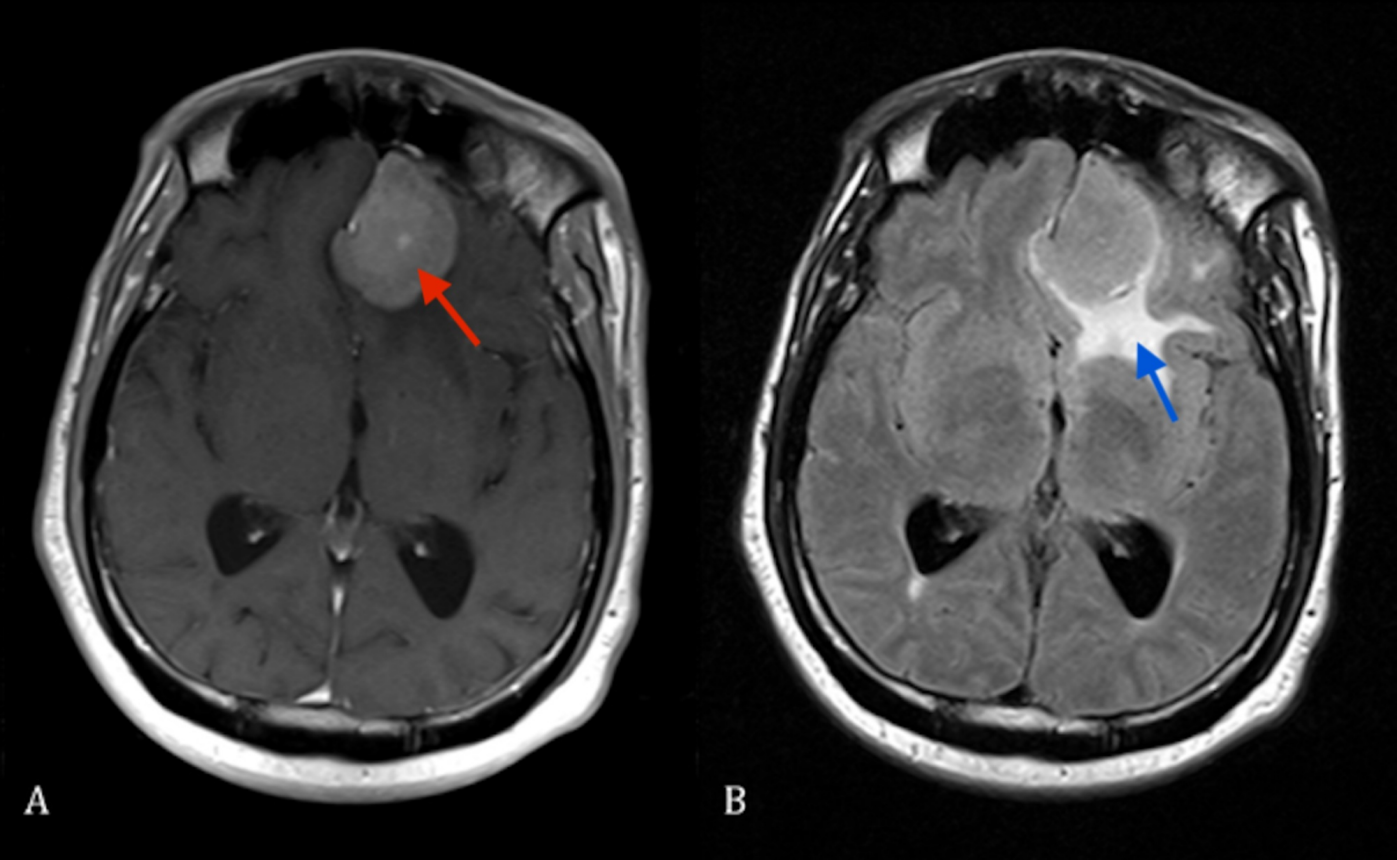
(A) Axial T1 MRI of brain with contrast showing left-sided olfactory groove meningioma (red arrow). (B) Axial FLAIR MRI of brain showing left-sided olfactory groove meningioma and surrounding edema (blue arrow). FLAIR: Fluid-attenuated inversion recovery; MRI: Magnetic resonance imaging.
